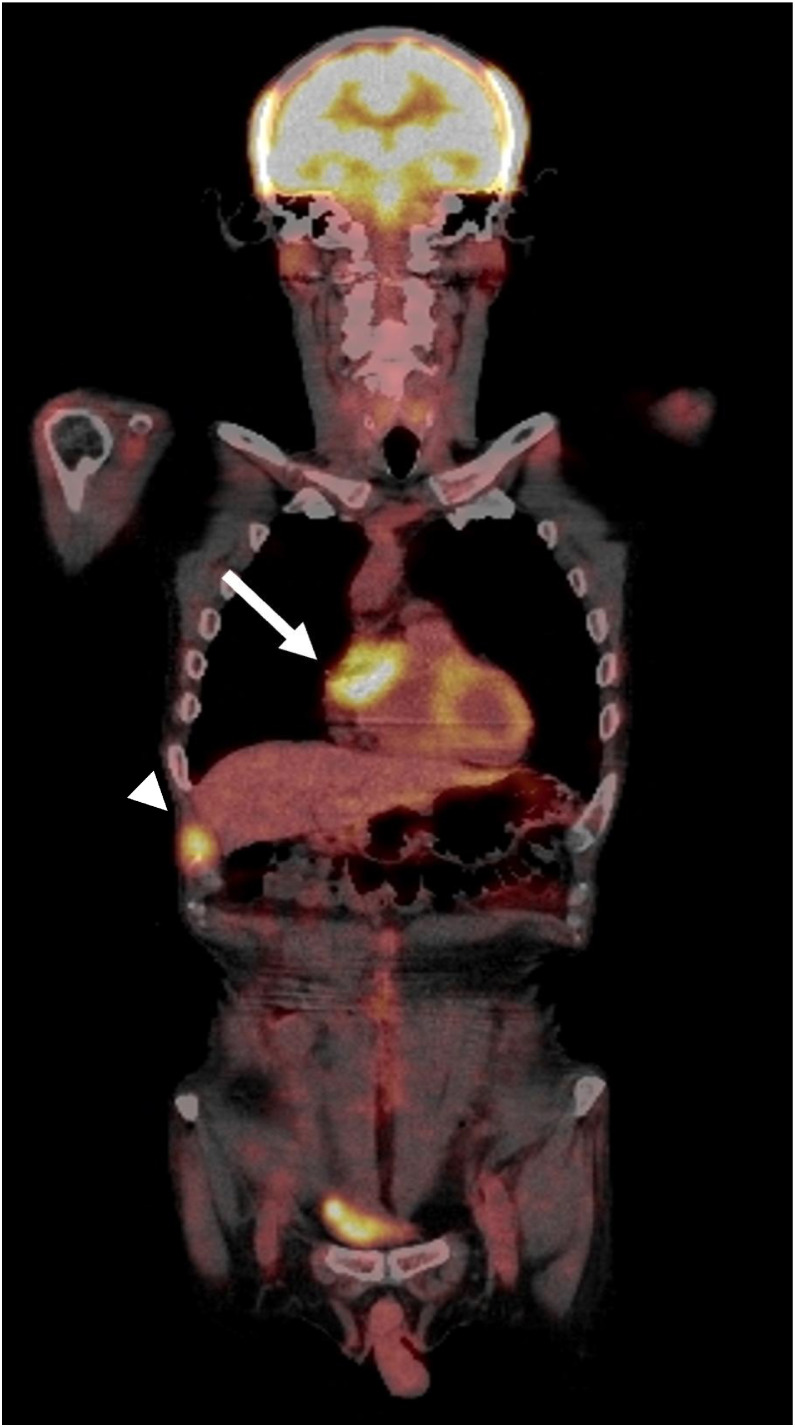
Positron emission tomography (PET)–computed tomography (CT) image 1 year after surgery. PET–CT image reveals 18F-fluorodeoxyglucose (FDG) uptakes in right atrial appendage (arrow, SUV_max_: 7.7) and right chest wall (arrowhead, SUV_max_: 6.3).
